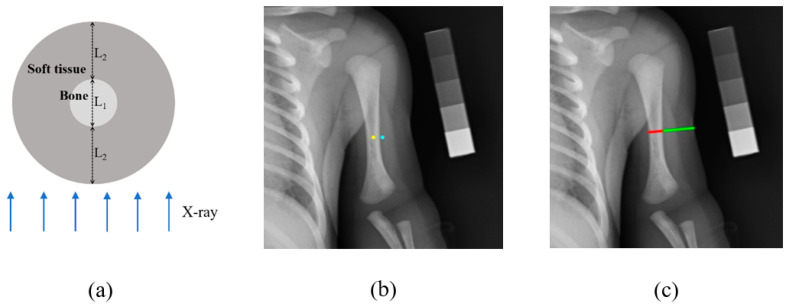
(a) The transverse view of the premature infant’s upper arm. (b) The yellow dot is a representative point of the bone area to be estimated, while the blue dot is a representative point of the soft tissue close to the bone. (c) Line segments are drawn on the X-ray image by an expert.
